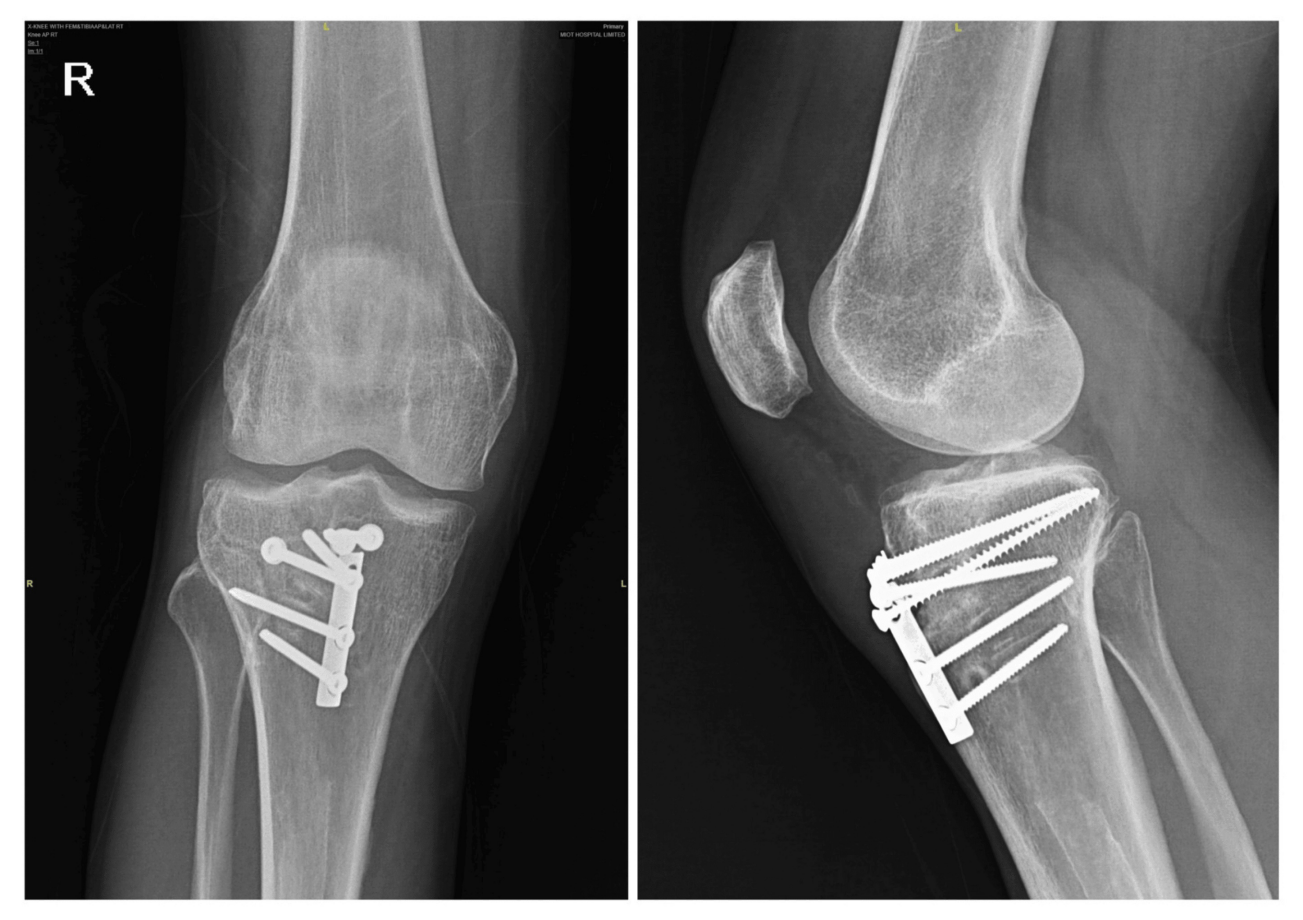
Four months' follow-up X-ray of the right knee showing a healed fracture after internal fixation
